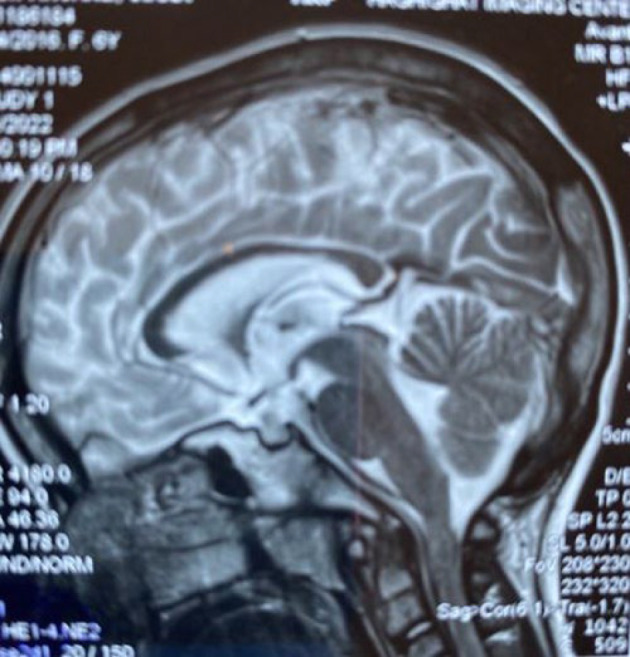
Female sibling sagittal T2 weighted brain MRI image shows thinning of corpus callosum
